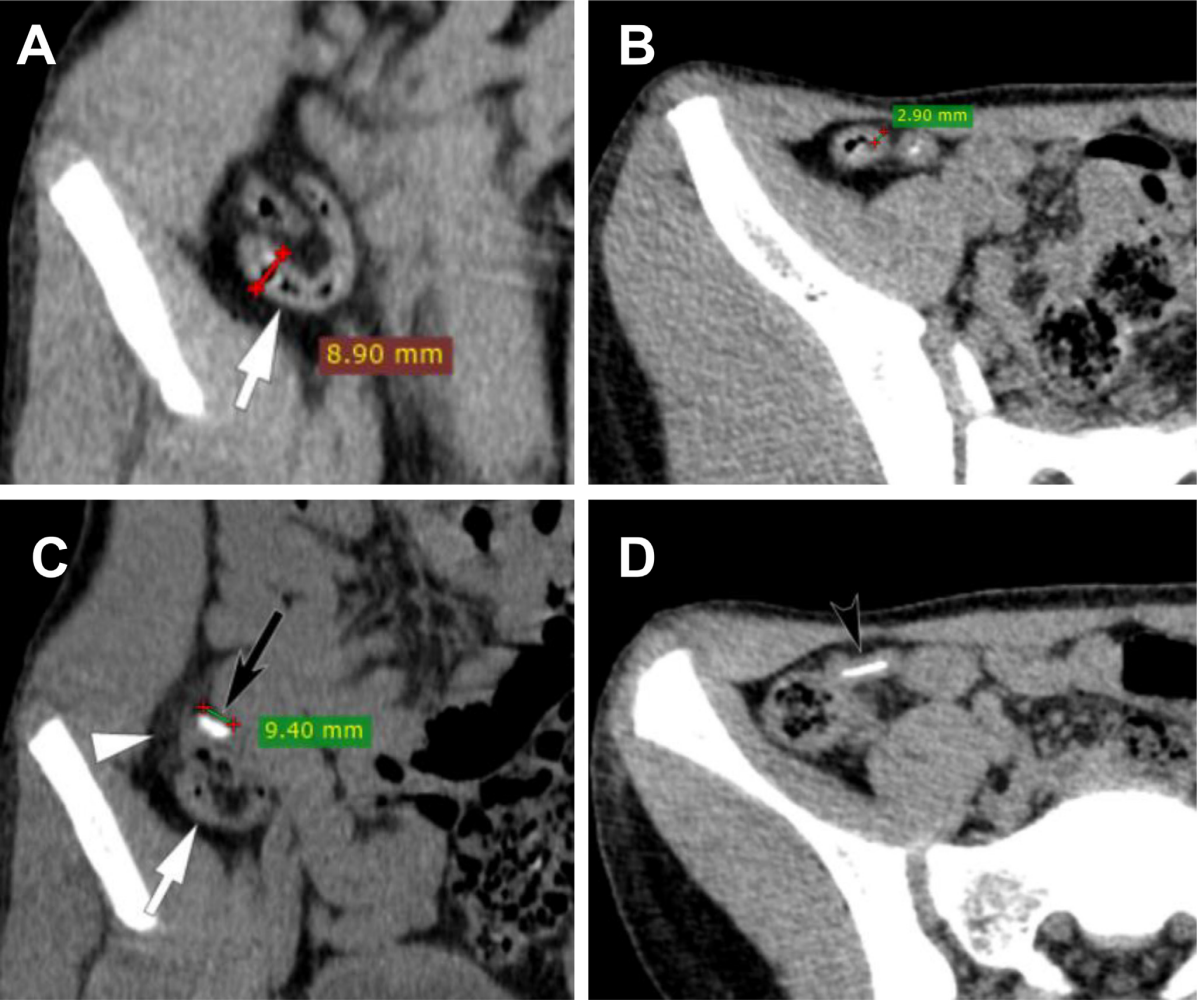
The abdominopelvic computed tomography images: (A, B) the appendix appeared enlarged, with a diameter of 8.9 mm and a wall thickness of 2.9 mm. (C) A radiopaque foreign body (black arrow), approximately 9.4 mm in length with well-defined edges, within the lumen of the appendix (white arrow). Axial CT image (C) also shows subtle fat stranding around the base of the appendix near a foreign object (white arrow head), in contrast to the body of the appendix, which shows no infiltration (black arrow head in image D).
